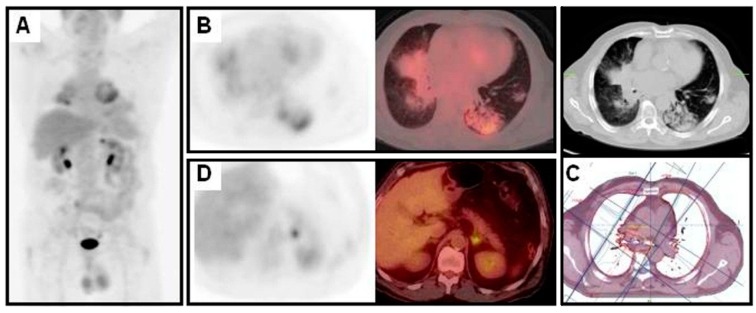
Patient with an adenocarcinoma near the right hilum treated with chemo-radiotherapy. A follow up CT performed four months later showed non-conclusive radiological lung findings. An ^18^F-FDG PET/CT showed increased lung uptake related to the radiotherapy area (A–C); Also, focal hypermetabolism in left adrenal gland was detected (D). Surgery confirmed an adrenal metastasis.
